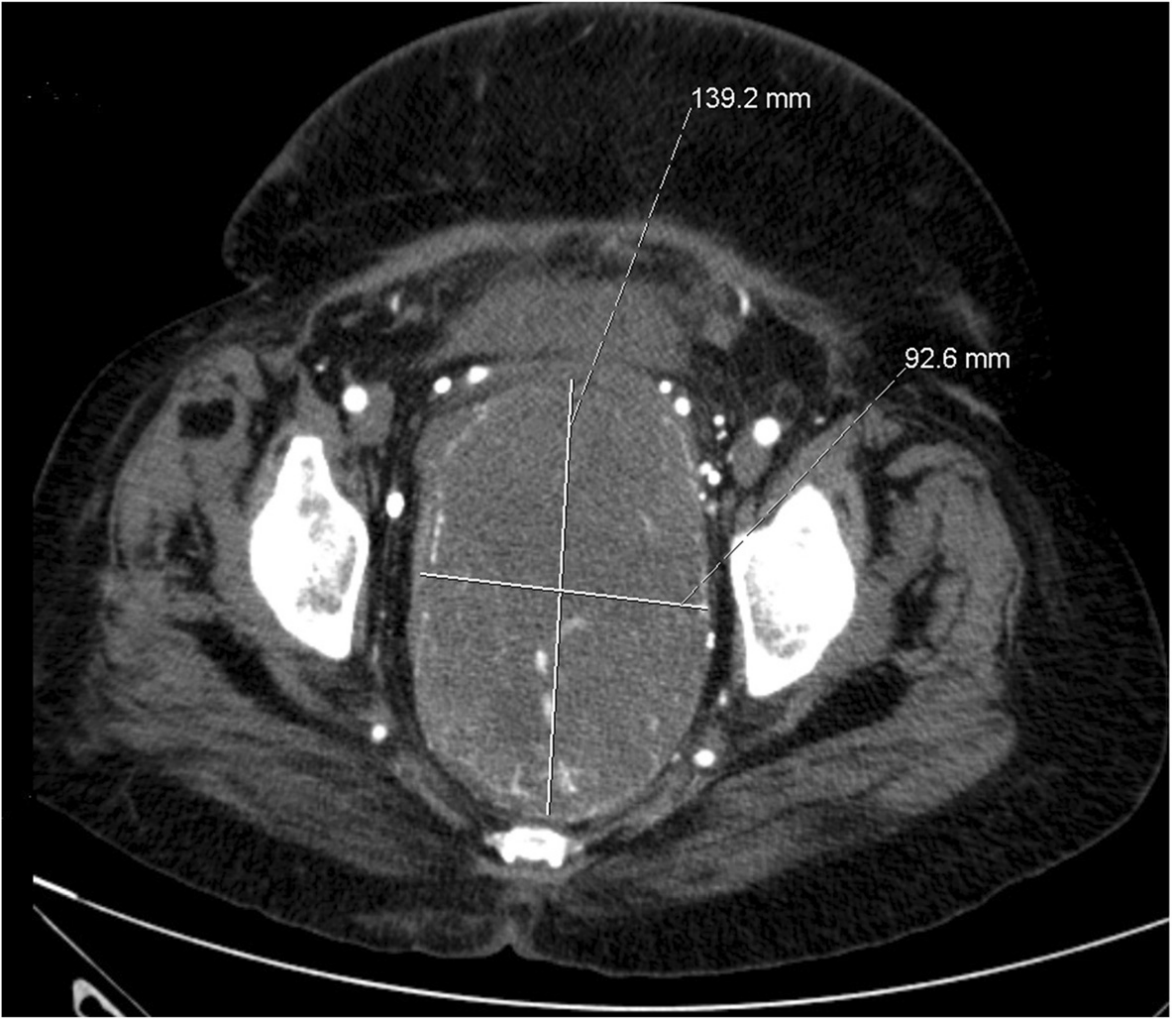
Preoperative CT showing the size, vascularization, and location of the tumor
